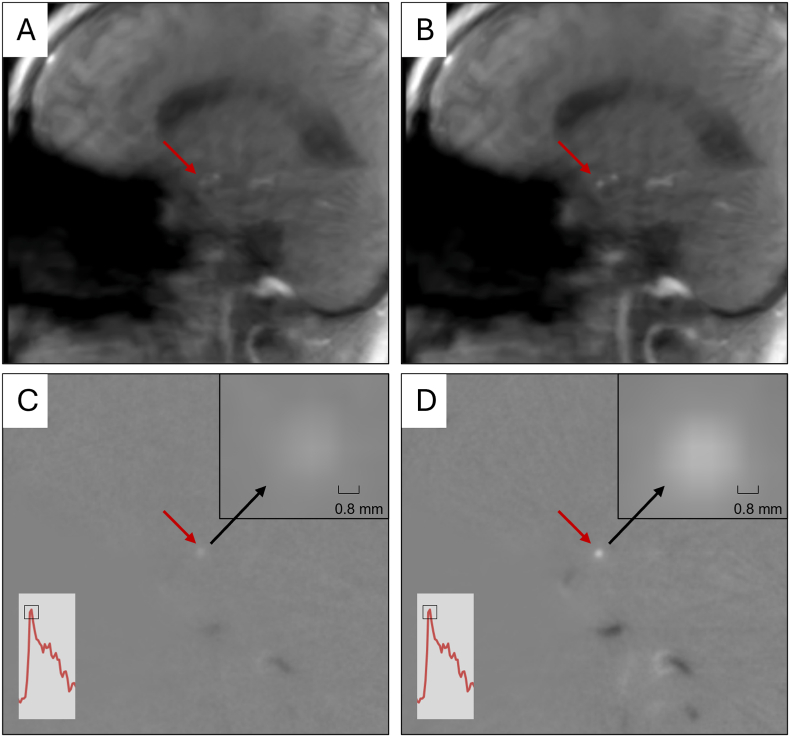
Magnitude- (A, B) and phase-contrast-images (C, D) from the RT-PC MRI during systolic phase of a single cardiac cycle at the end of hyperventilation (A, C) and at the end of apnea (B, D) in CVRC-testing of the MCA. The signal of the MCA is more hyperintense during the apnea-phase (B, D) compared to the hyperventilation-phase (A, C), because of vasodilation and therefore increased blood flow. Furthermore, the MCA during hyperventilation (C) and apnea (D) in phase-contrast images is represented in detailed zoomed-in view. The predefined area of the ROI during this measurement was 5.76 mm^2^. Both magnitude- and phase-contrast-images represent the systolic phase of one cardiac cycle at the end of hyperventilation and at the end of apnea.
